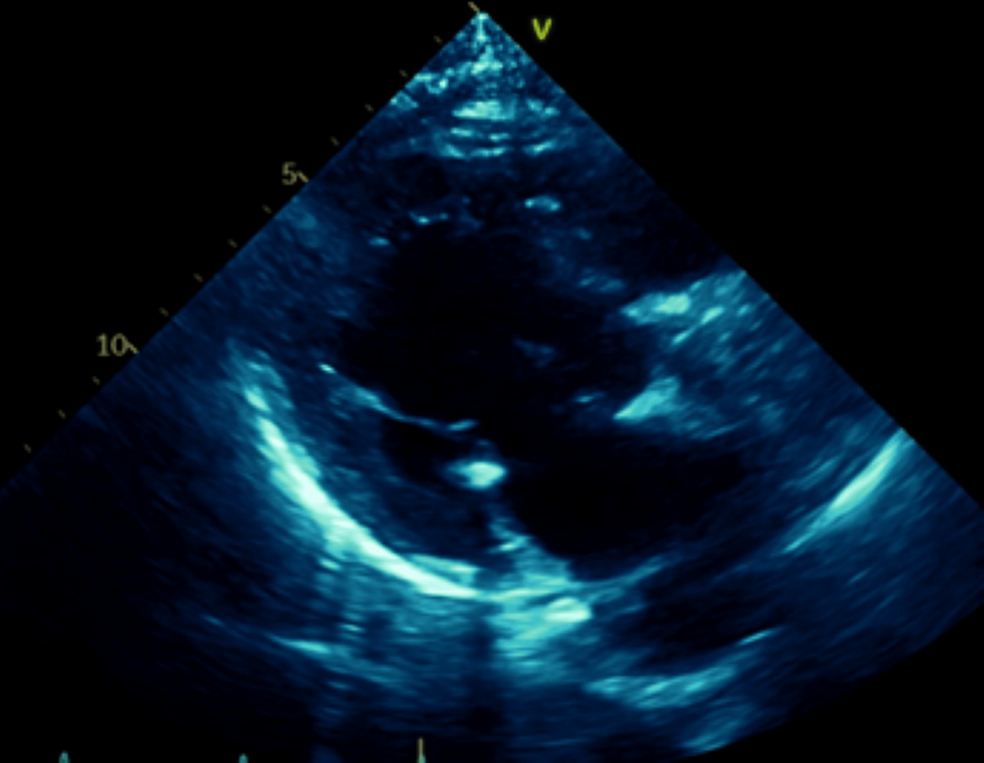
Eight Weeks Post TAVR. Thrombosed Valve while on Apixaban Ultrasound parasternal long-axis view showing thickening of bioprosthetic valve leaflets. TAVR: transcatheter aortic valve replacement
